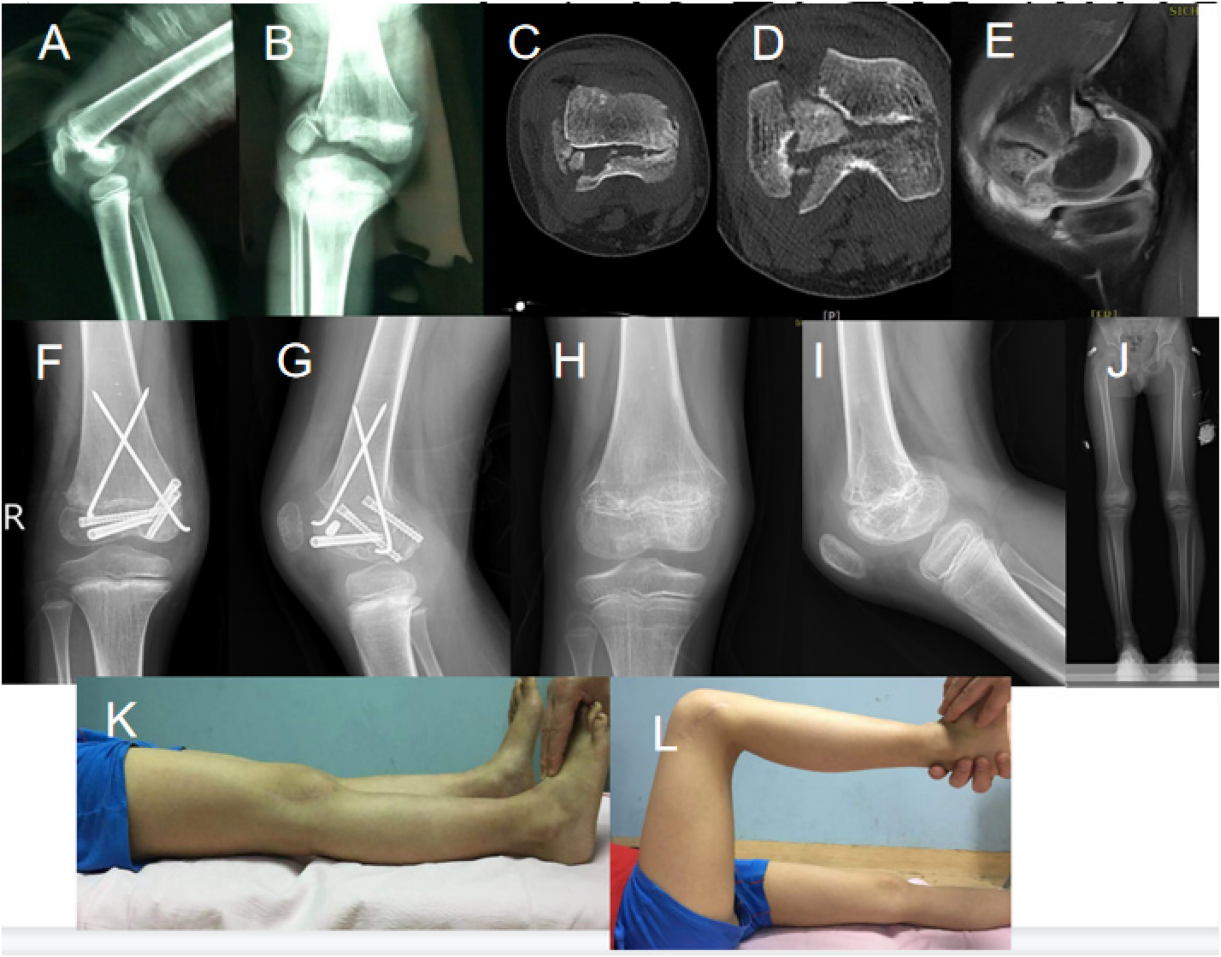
An injured 7.9-year-old boy with bicondylar Hoffa's fracture arising from a high fall injury. (A, B) X-rays of the fracture taken in a preoperative state; (C, D, E) CT and MRI performed in a preoperative state and establishing the injury severity; (F, G) Post-operative X-rays showing internal fixation; (H, I) X-rays demonstrating bone healing during follow-up; (J) full-length X-ray of both lower limbs; (K, L) clinical images showing functional recovery and range of motion.
